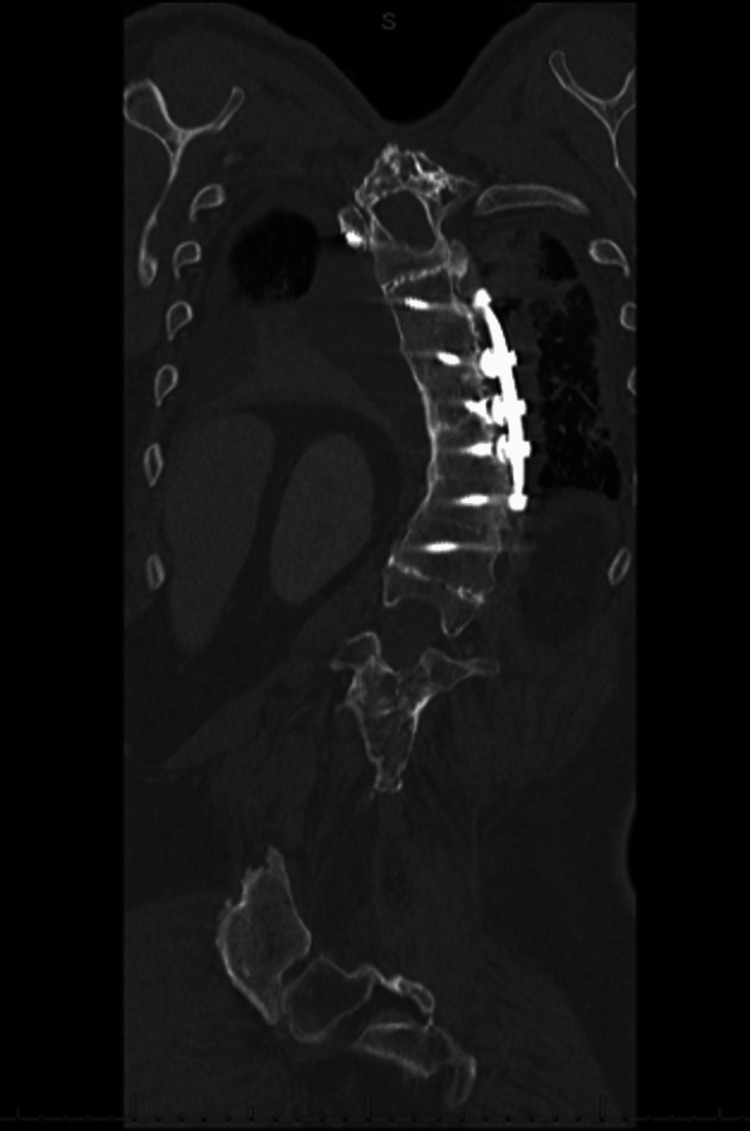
CTA of the chest revealing intact fusion hardware close to the left lung (coronal view) CTA: computed tomography angiogram
